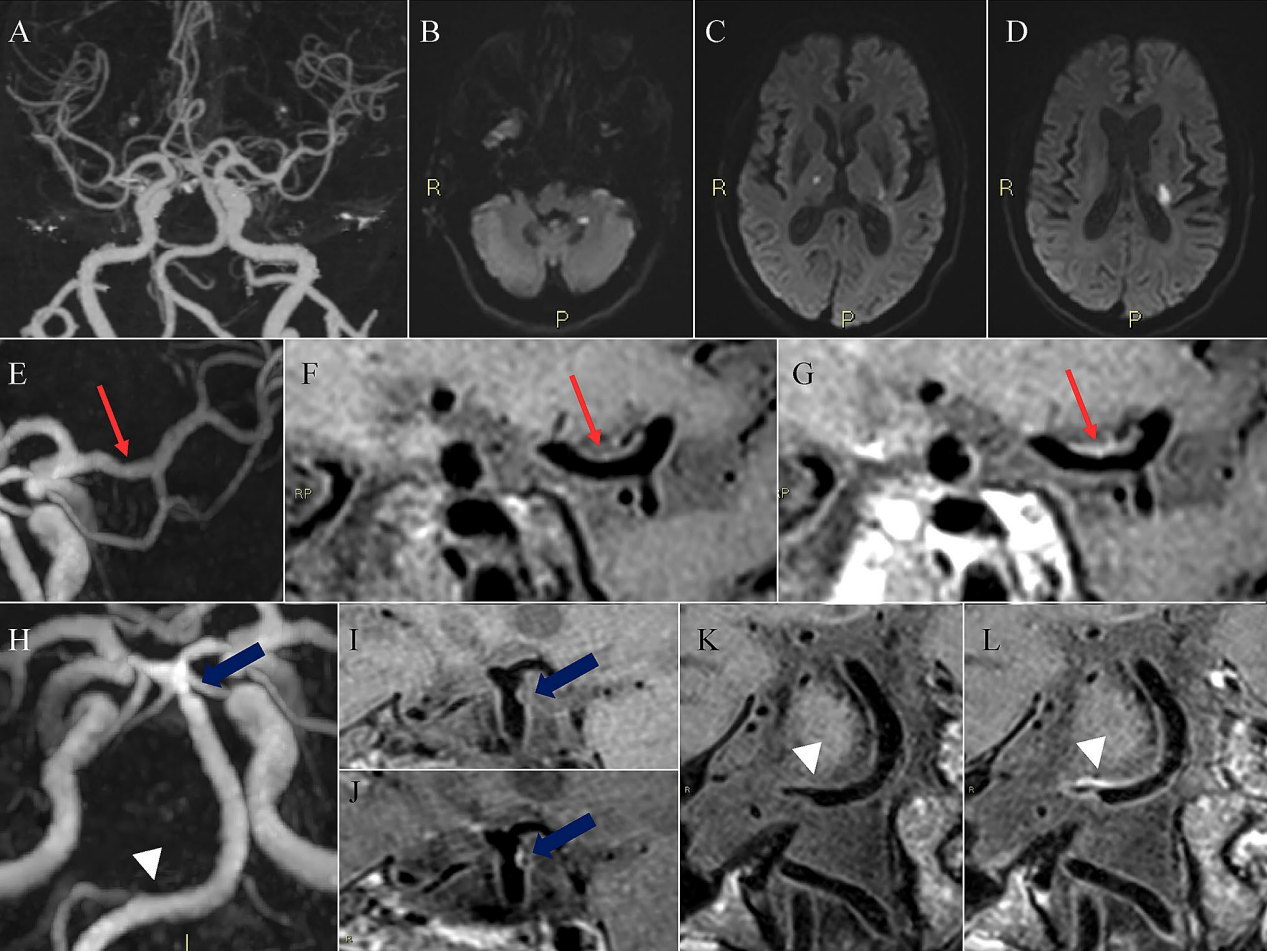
CNS vasculitis. A 58-year-old man with dizziness, vomiting, and speech disturbance underwent MRI study demonstrating in the TOF sequence, the presence of multiple caliber alterations in the intracranial arterial circulation (A), associated with recent ischemic lesions in the brain tissue (B–D) both in the anterior and in the posterior circulation. The caliber alterations detected in the TOF sequence demonstrated the presence of wall enhancement after the administration of contrast medium (G, J, l), particularly in the left M1 segment of the middle cerebral artery (E, F, G, red arrows), at the basilar artery apex (H, I, J blue arrows) and in the proximal portion of the basilar artery at the level of the right anterior inferior cerebellar artery (H, K, L white arrowheads). The laboratory test demonstrated the positivity for a T. pallidum infection and thus the final diagnosis is a luetic CNS vasculitis
